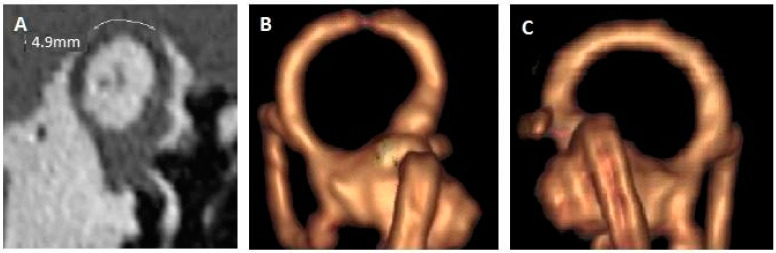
Left SSCD with partial auto-plugging. (A) HRCT, Pöschl plane; dehiscence measured at 4.9 mm. (B) 3D HR MRI, reconstruction in the plane of the SSC (left ear). (C) 3D HR MRI, reconstruction of the labyrinth, right ear; the image is provided for comparison. MRI: magnetic resonance imaging; HRCT: high resolution cranial tomodensitometry; SSCD: superior semicircular canal dehiscence.
